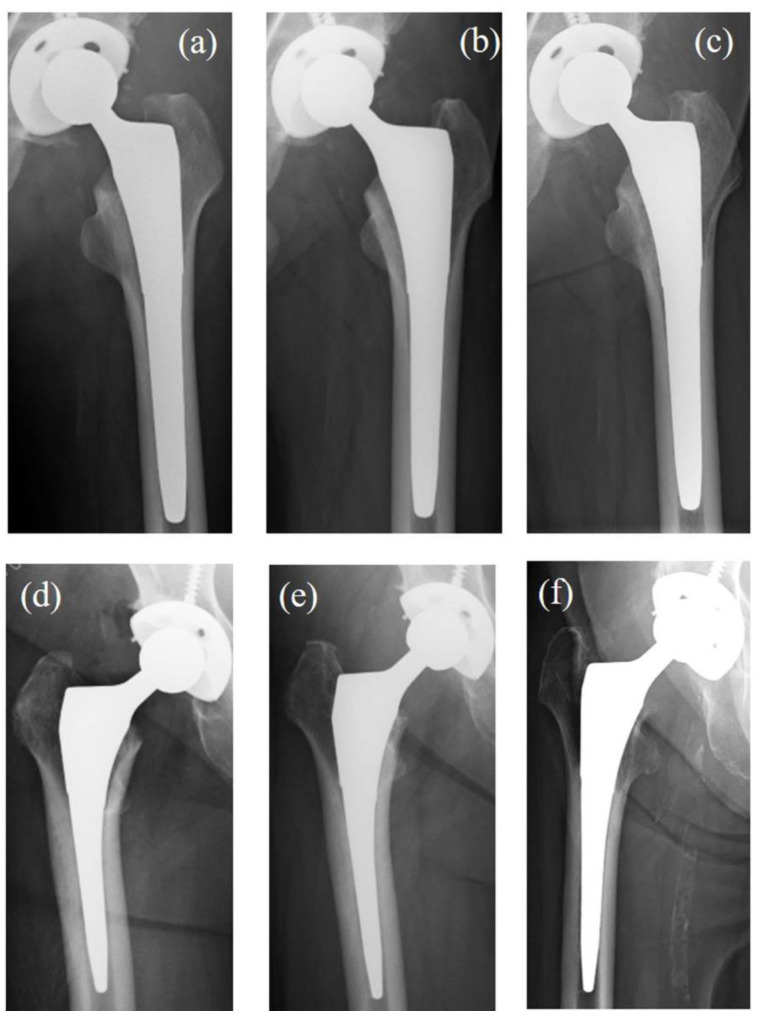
The X-rays of two cases of THA were performed using a TNS stem. Case 1 (a–c): A 58-year-old male diagnosed with idiopathic osteonecrosis of the left femoral head. No SS was observed at the final follow-up. (a) X-ray 3 weeks post operation. (b) X-ray 52 weeks post operation. (c) X-ray 94 months post operation. Case 2 (d–f): A 68-year-old female diagnosed with right hip osteoarthritis. Progressive SS was observed over time. (d) X-ray 3 weeks post operation. (e) X-ray 52 weeks post operation, showing rounding of the proximal femoral neck, consistent with first SS. (f) X-ray 85 months post operation, showing medial cortical bone resorption extending below the lesser trochanter, consistent with third SS. THA: total hip arthroplasty. SS: stress shielding.
